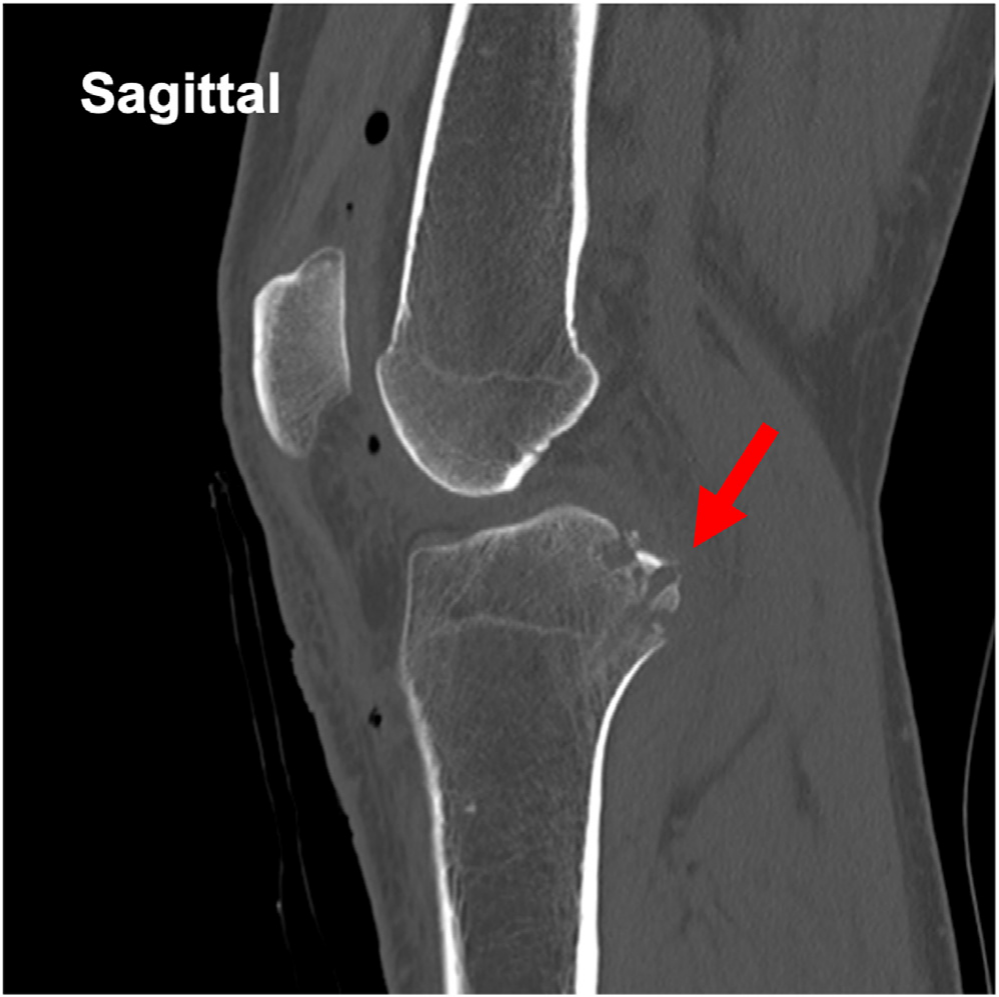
Postoperative sagittal computed tomography in the right knee.Anatomic reduction is obtained (arrow).
